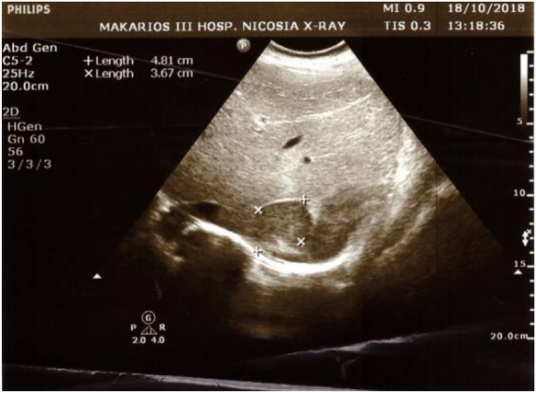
Ultrasound image of the liver and adrenal mass x/+ indicating dimensions
